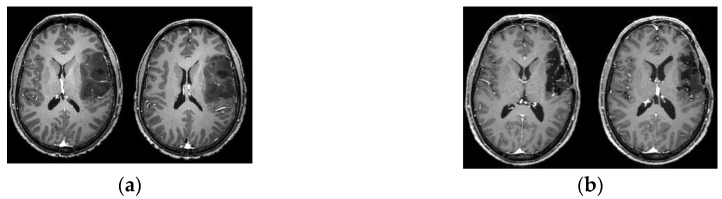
Axial view T1-weighted MRI. Upper image shows frontotemporoinsular glioma before first surgery. (a) Lower images show recurrence before second surgery. (b) Recurrent neoplastic tissue was located both along the walls of and within the previous-tumor cavity.
